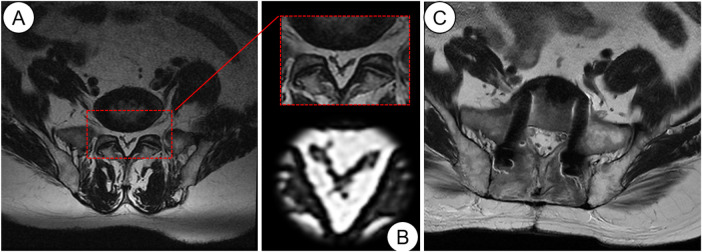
(A) accumulation of fat within the lumbar spinal canal on lumbar spine MRI T2; (B) is an enlarged view of panel (A), showing a “V” shaped compression of the dura mater; (C) postoperative lumbar spine MRI indicating the compressed dura mater has returned to its original shape.
